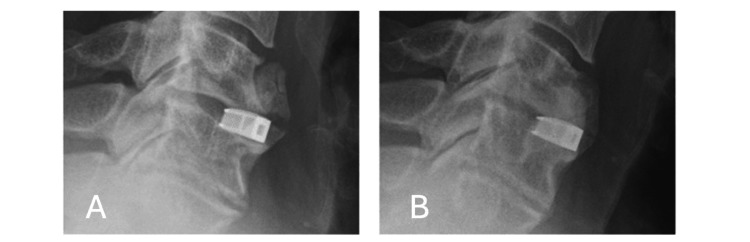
A case showing ideal vertebral body fusion A. Day one post-operative x-ray showing the implant in the intervertebral space B. Follow-up x-ray showing vertebral body fusion and osteointegration of the titanium implant.
